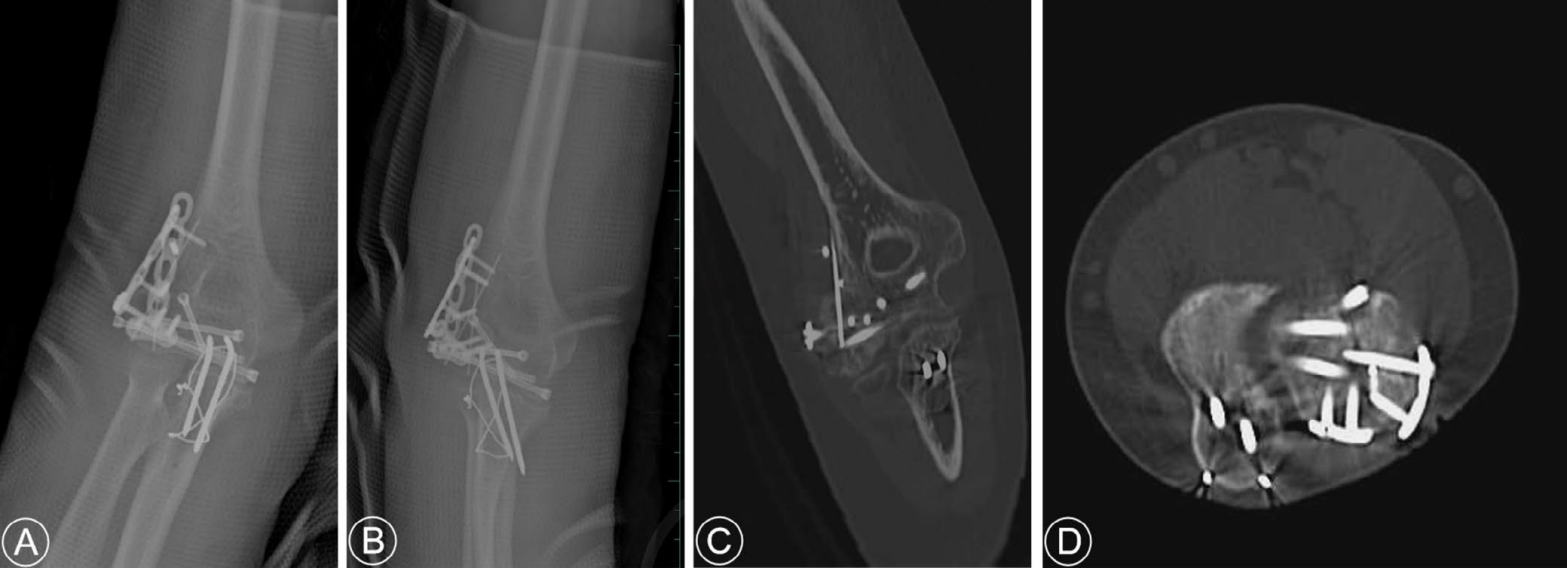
Postoperative image. (A, B) Two pictures of postoperative x‐rays. (C, D) Coronal and sagittal images in CT scans.
